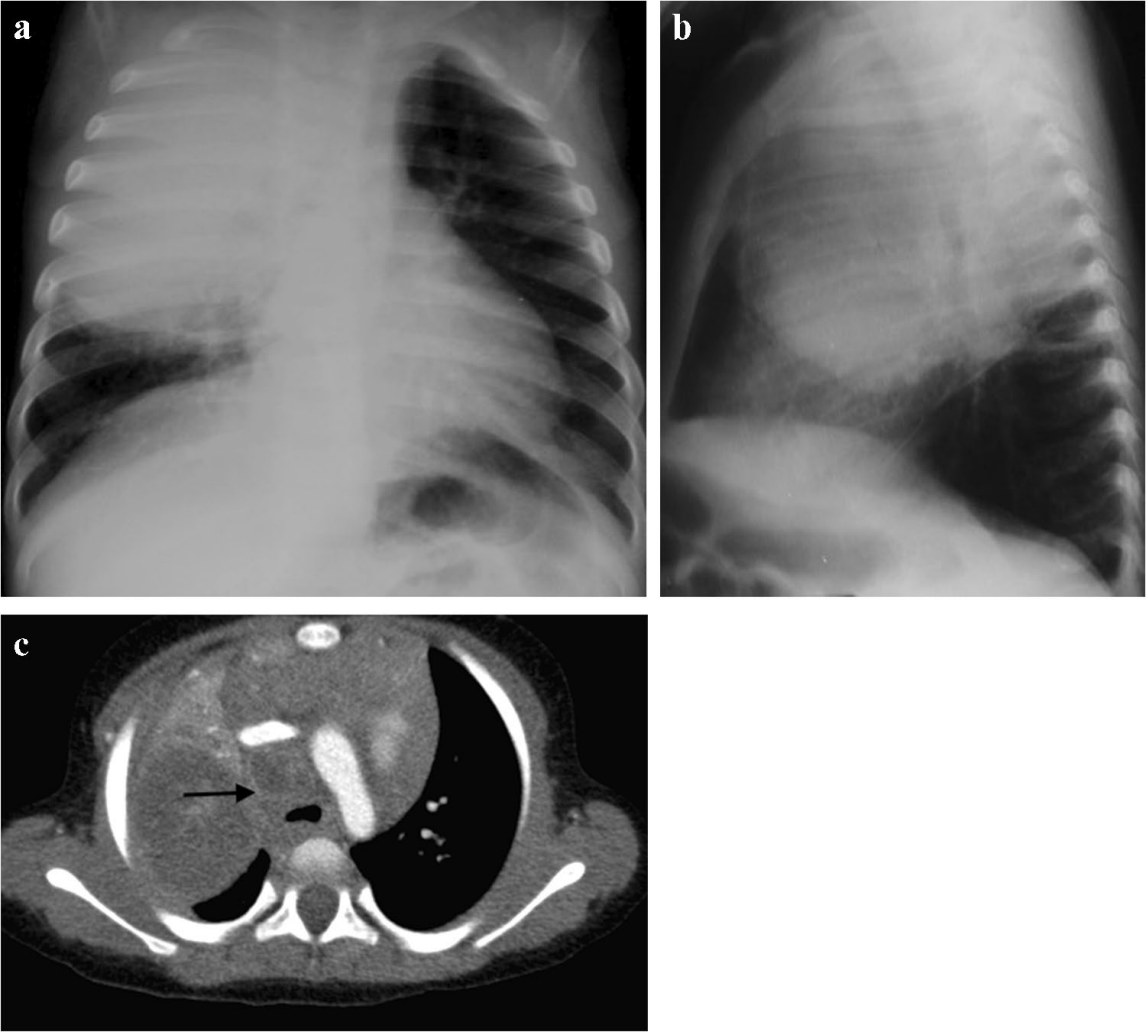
Chest imaging in a 2-year-old girl with pulmonary tuberculosis. a Posteroanterior chest radiograph (CXR) shows pneumonia of the right upper lobe. Note the displacement of the trachea towards the left and attenuation of the bronchus intermedius suggesting paratracheal lymphadenopathy. b Lateral CXR shows inferior bulging of the horizontal fissure and soft tissue density of lymphadenopathy around the carina. c A post-contrast axial computed tomography image (mediastinal window) demonstrates large volume lymphadenopathy of low density and peripherally enhancing lymph nodes (*arrow*). The right upper lobe is expanded and of predominantly low density, consistent with caseous necrosis. There is a small associated pleural effusion
